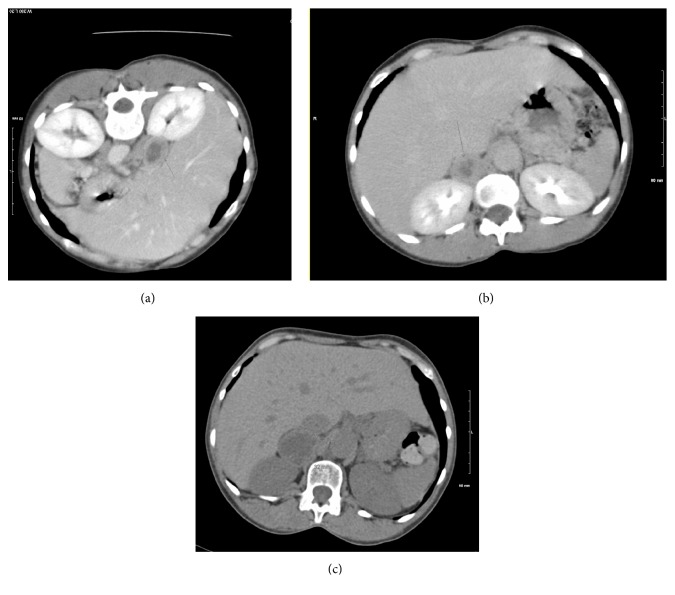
(a-b) CT abdomen and pelvis with contrast in 2006 demonstrating a large right adrenal mass with a hypodense center (a) venous phase and (b) delayed imaging. (c) Noncontrast CT abdomen pelvis in 2013 showing interval increase in size of the right adrenal mass to 3.7 x 3.2 cm from 2.3 x 1.9 cm.
